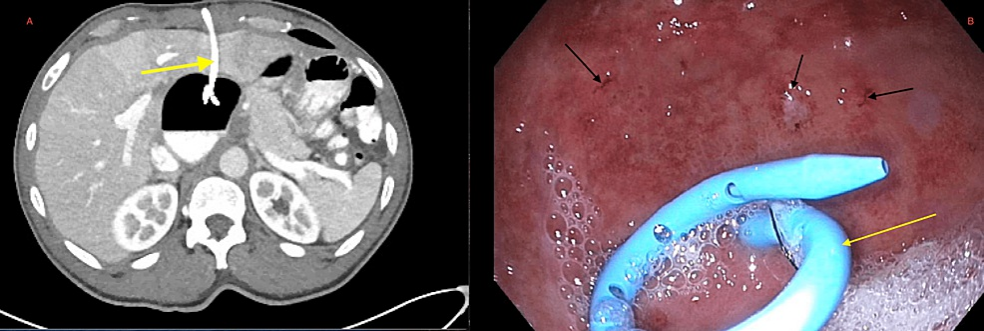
Yellow arrows show the percutaneous drain visualized in the gastric body on CT scan (A) and on the EGD (B). Gastric body ulcers are highlighted by black arrows (B). EGD: esophagogastroduodenoscopy
